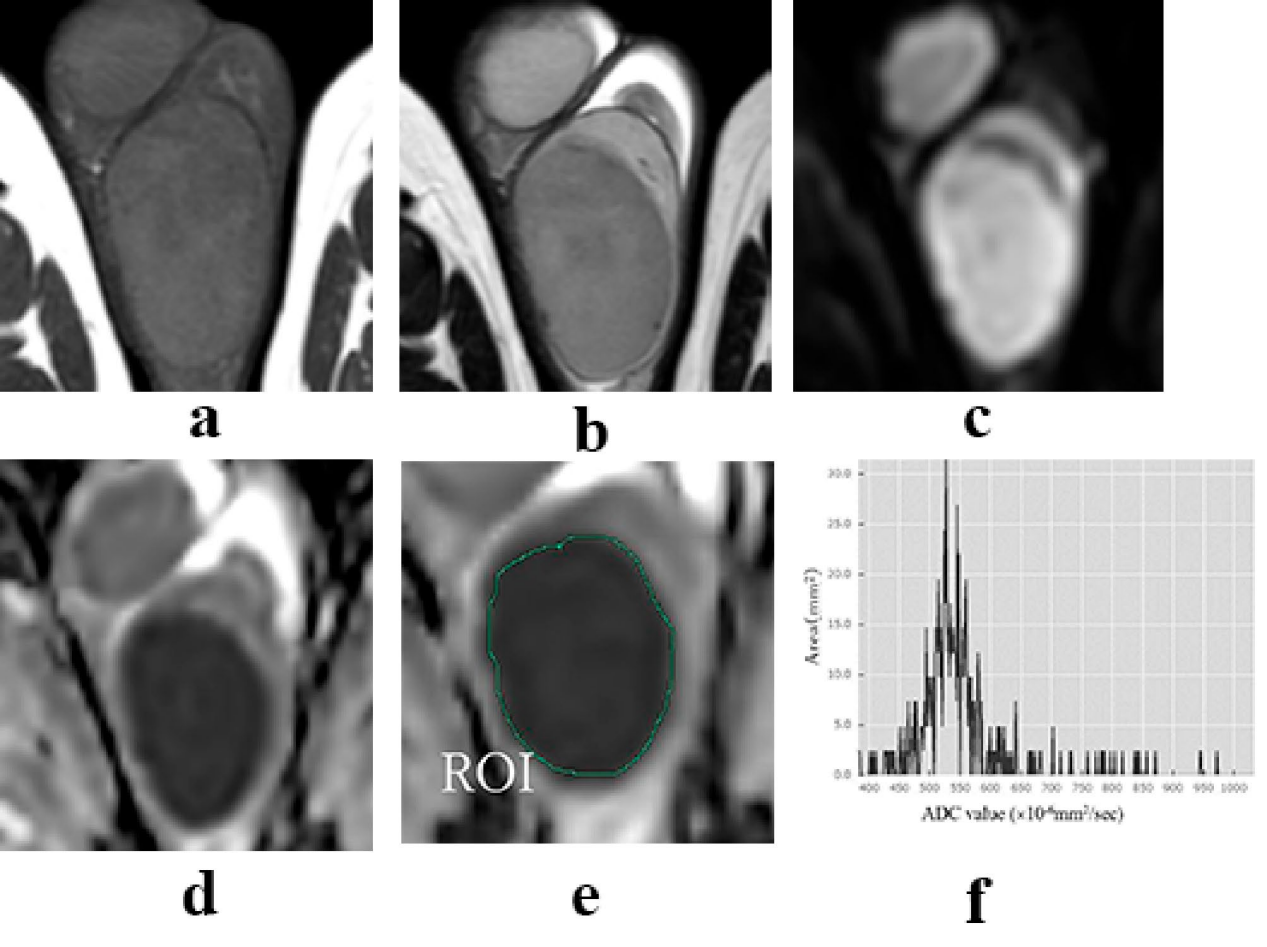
Representative findings of malignant lymphoma. T1W1(a), T2W1(b), DWI (c), and ADC map (d). The ROI surrounded the entire tumor on the ADC map (e). Histogram of the ADC value (f). The histogram of the seminoma showed high kurtosis and skewness values of 15.520 and 2.929, respectively. It had a shape with peaked peaks and heavy fringes compared with the normal distribution. The overall distribution of ADC values was skewed toward lower values than that of seminoma. T1WI: T1-weighted imaging, T2WI: T2-weighted imaging, ADC: apparent diffusion coefficient, ROI: region of interest, DWI: diffusion-weighted imaging
